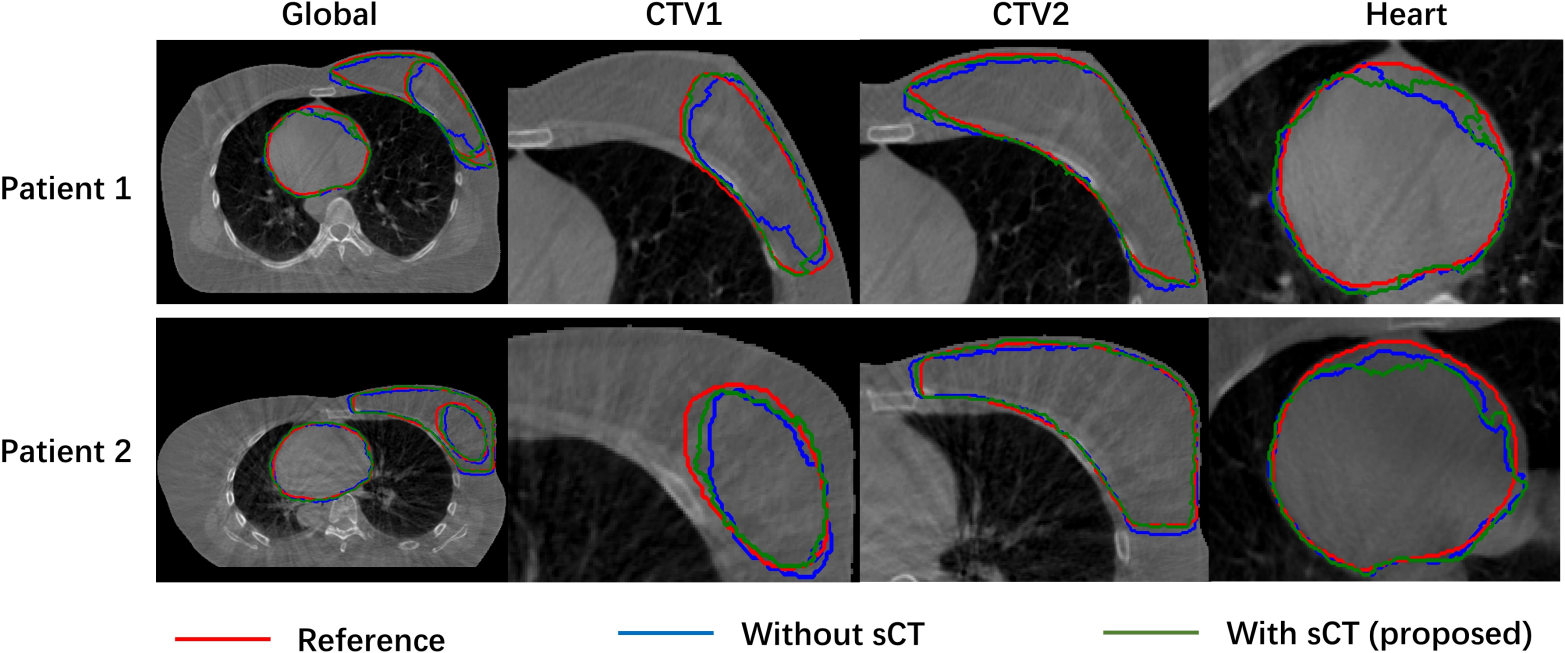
Comparison of object segmentation performance of fixed image using CBCT image and sCT image.
